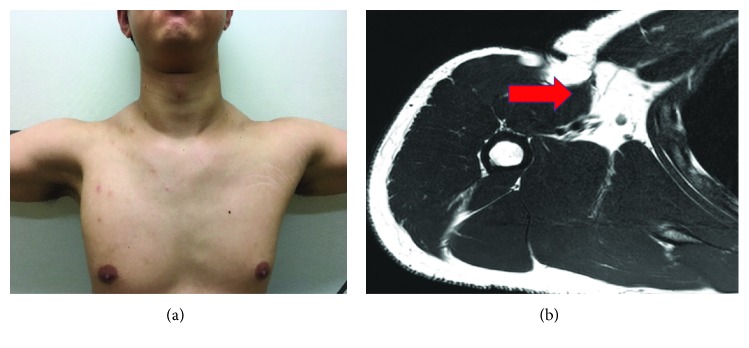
(a) The right shoulder presented with loss of axilla fold with no bruises due to chronic presentation. (b) T2-weighted images of MRI showed complete torn of pectoralis major muscle in the osteotendinous insertion with retraction up to the medial border of the anterior margin of the deltoid muscle.
